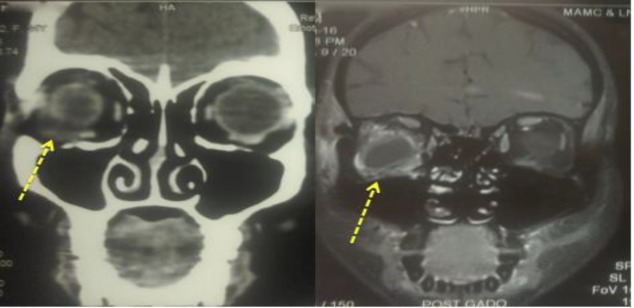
Coronal cuts of CECT and MRI with cystic swelling; likely an abscess in the right orbit
